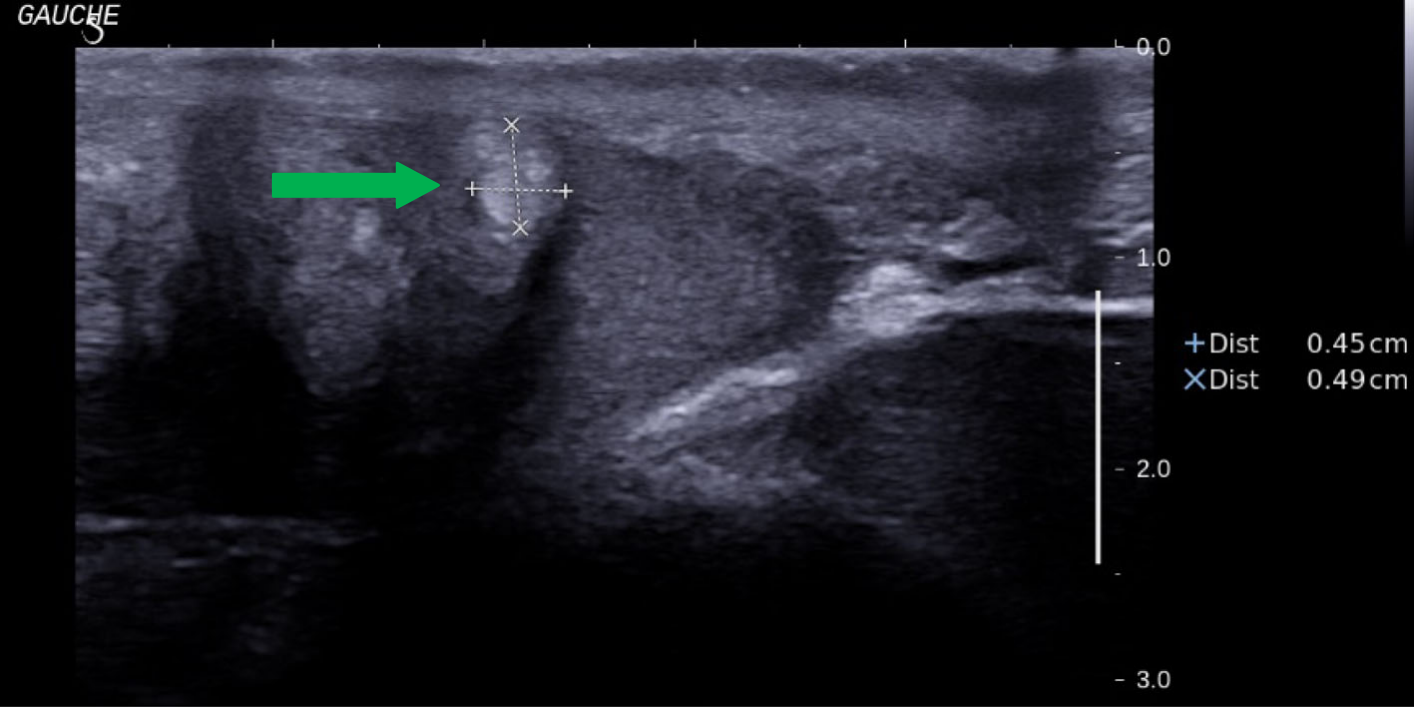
Scrotal ultrasounds of the left testis from second case. Legend. Arrow indicates Testicular Adrenal Rest Tumors (TART)
